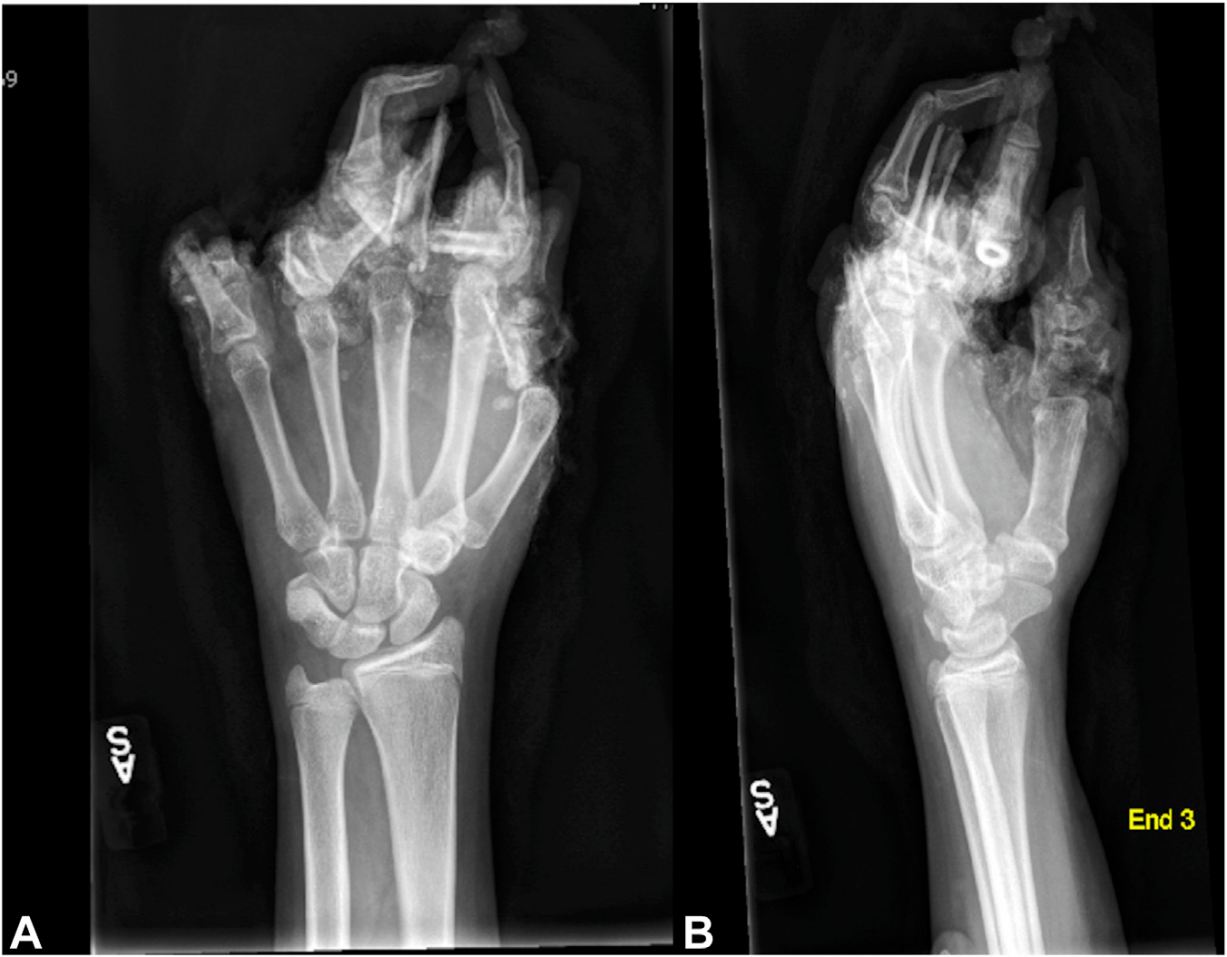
These radiographs are of a 12-year-old girl who sustained multiple open fractures after a UTV ROE. The patient was riding passenger, directly behind the driver in a four-seater UTV. During the ROE, the patient stuck her hand outside the vehicle to brace for impact. Amputations to the thumb, index, middle, and small fingers were performed as replantation was unfeasible due the extensive crush-avulsion trauma to the soft tissues.
